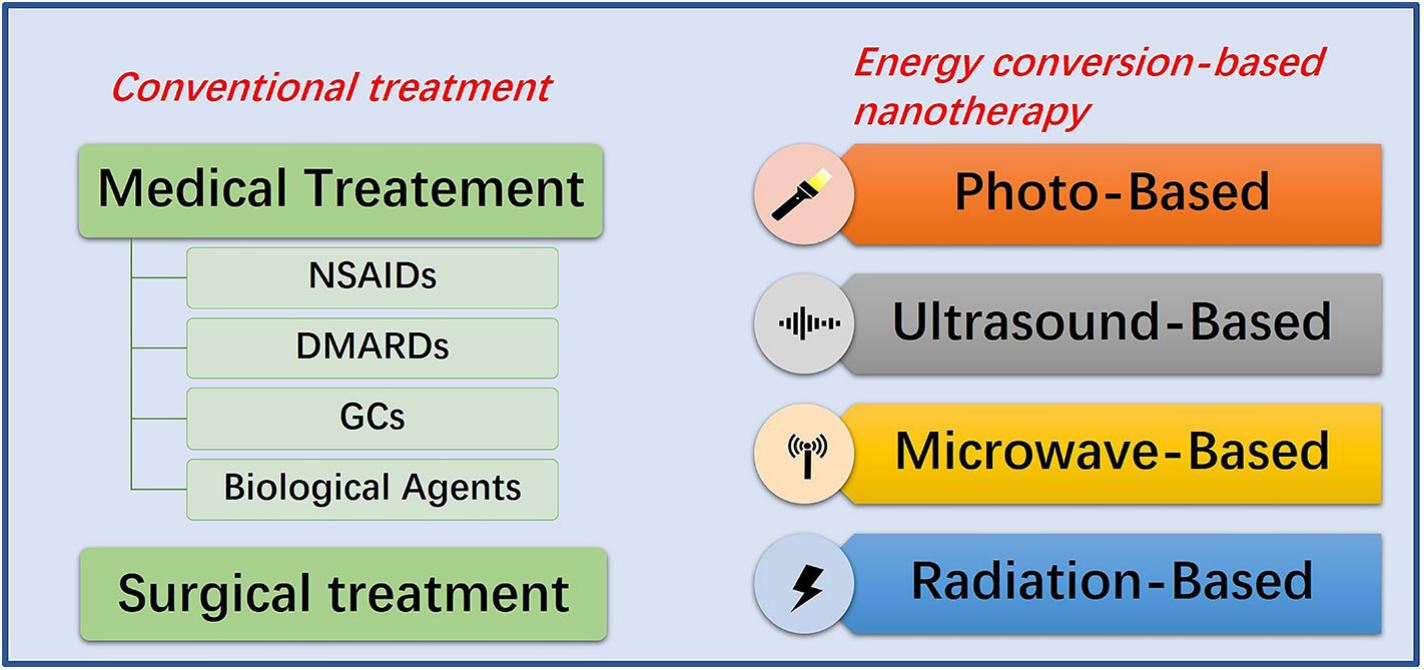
Summative scheme of conventional treatment strategies and energy conversion-based nanotherapies for RA treatment. Conventional treatment strategies include medication and surgery. Energy conversion-based nanotherapies include photo-based, ultrasound-based, microwave-based and radiation-based nanotherapy.
